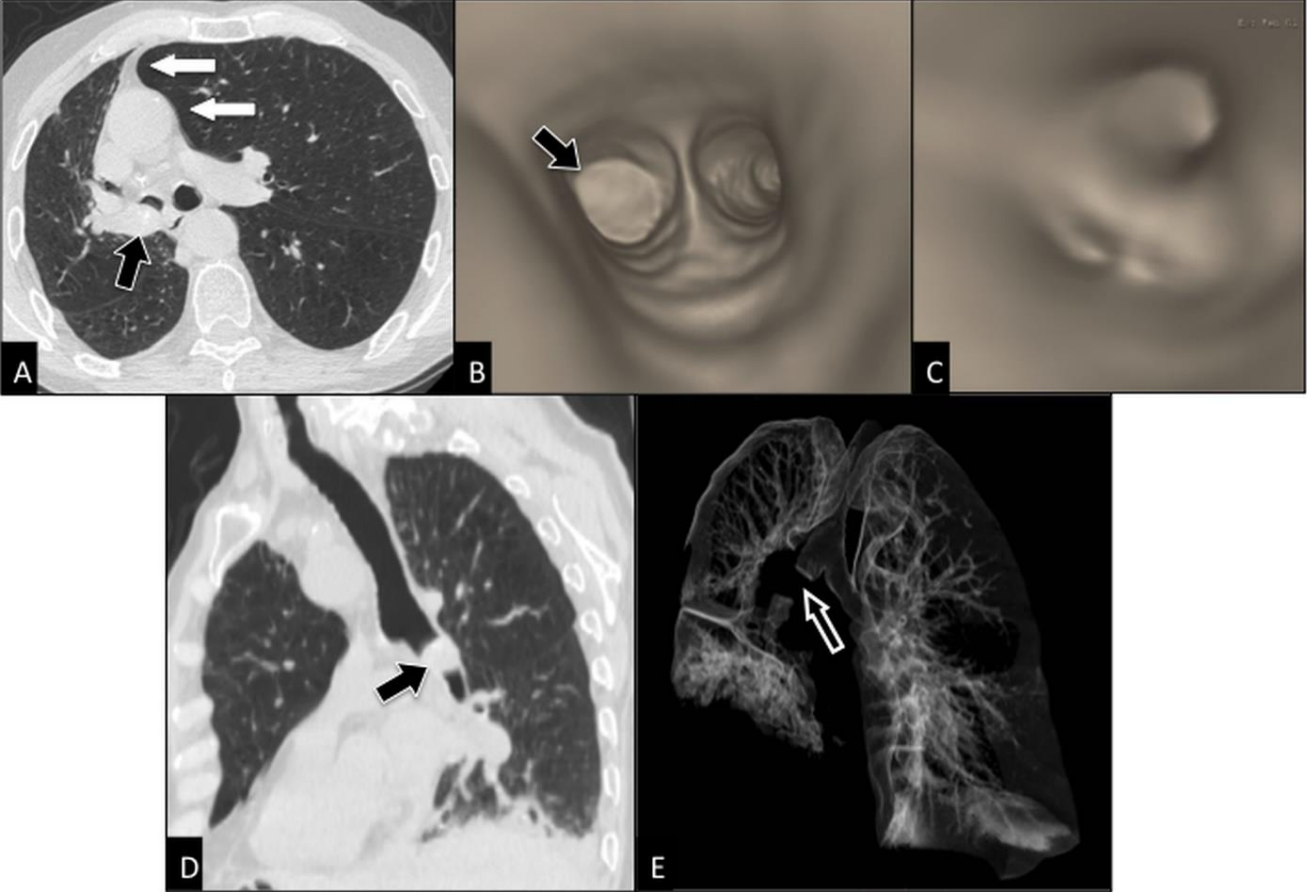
Post-processing imaging techniques: 2D and 3D reconstruction images Axial CT scan (A) shows an endobronchial mass *(black arrow)* within right main bronchus in a 78 years-old man with squamous cell carcinoma, causing partial collapse of right lung with displacement of mediastinum towards right *(white arrows)*. 3D virtual bronchoscopy (B, C) reproduces the bronchoscopic appearance of the mass occluding right main bronchus *(arrow in b)*, but it allows more than bronchoscopy the visualization of the patent bronchi distal to obstruction site (C). Curved 2D MPR (multiplanar reconstructions) image (D) better depicts the cranio-caudal extent of the mass *(arrow)* into right main bronchus than the axial image (A). CT bronchography (E) allows a global visualization of the aerated lung and shows the site of interruption *(arrow)* of the tracheobronchial tree.
